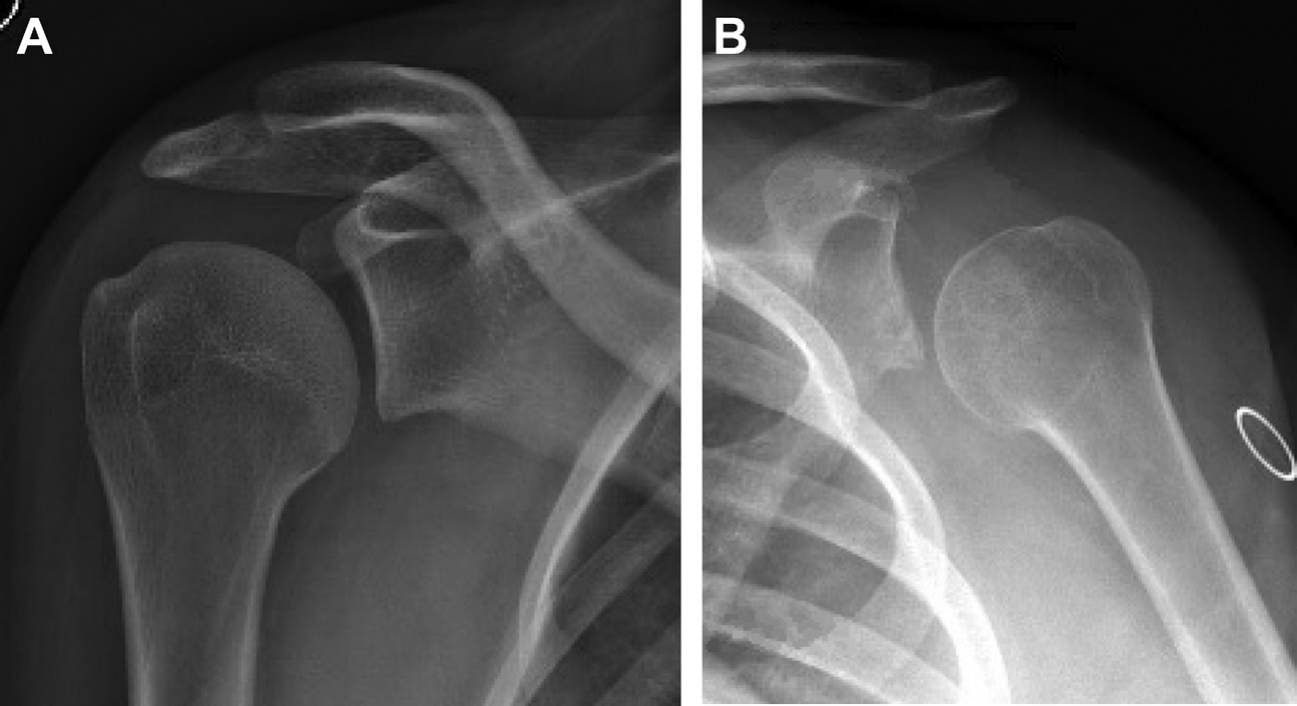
Radiographic demonstration of inferior subluxation of the humeral head in hEDS on Grashey views in patients with mild (A) and severe capsule laxity (B), indicating poor capsular integrity.
